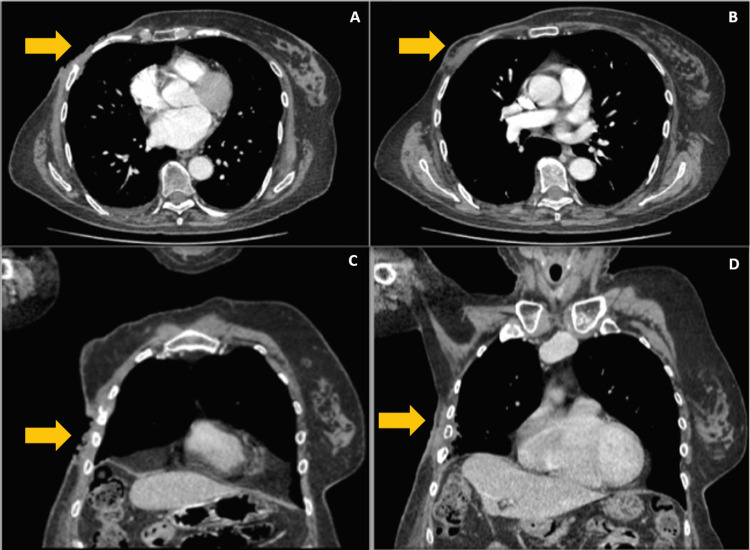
Contrasted CT scan of the thorax shows the mass on the right breast and costal archs (A-B, axial view; C-D, coronal view) The yellow arrow points the area of depth of the mass.
